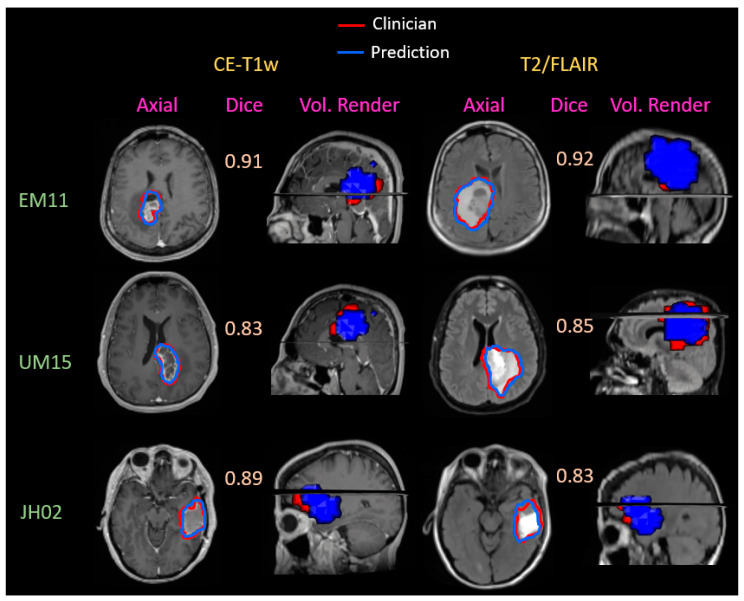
Example GTV2 (CE-T1w target) and GTV1 (T2w/FLAIR target) segmentations for patients from three different institutions with our best-performing 3D Unet model. For GTV2 and GTV1, we have included an axial view of the clinician GTVs (red) and the prediction (blue) as well as a volume-rendered view of the contour overlap in the sagittal orientation. Dice scores are included between the 2D and volume rendering for each example.
